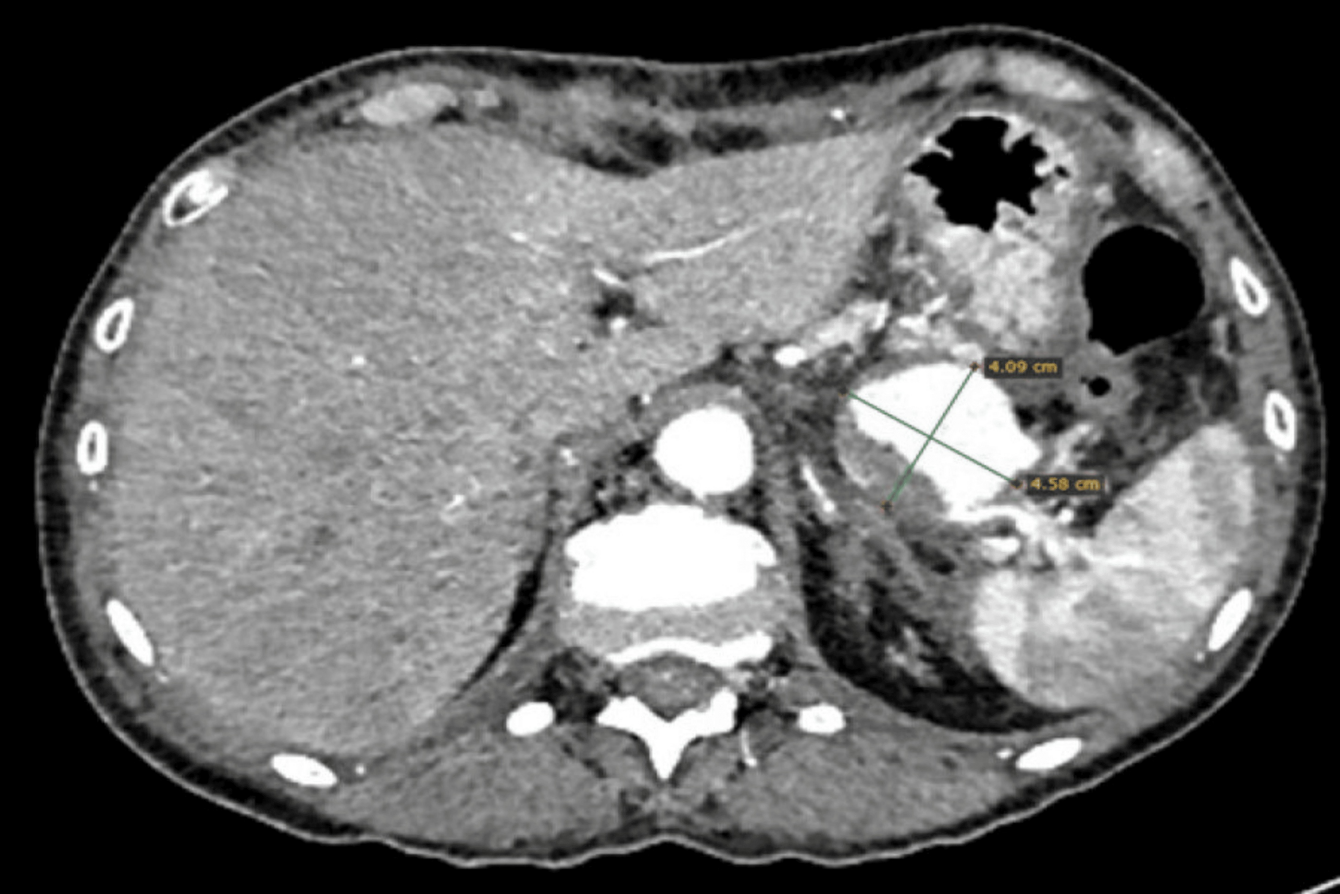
CTA demonstrating a pseudoaneurysm of the distal splenic artery. Multiple peripheral splenic infarcts are evident. CTA: CT angiography
